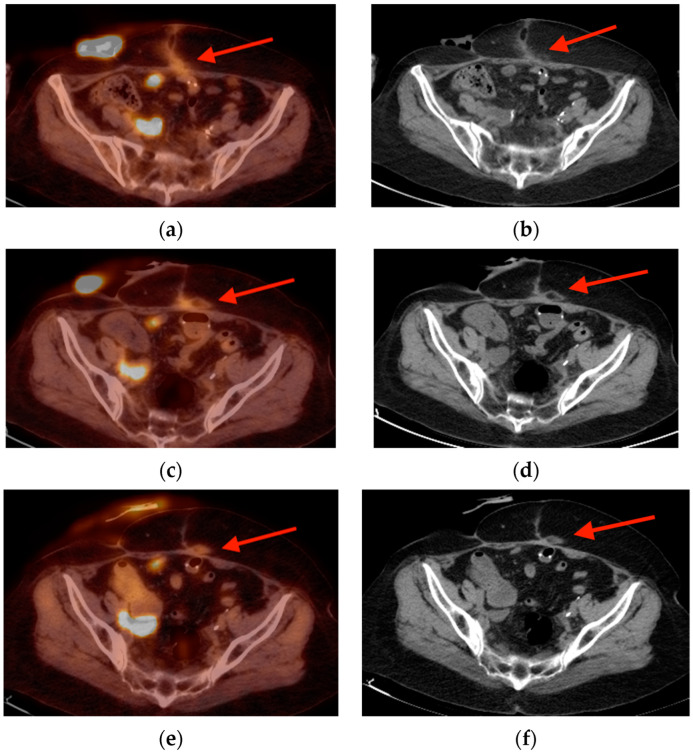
Multiple scans were obtained in a 72-year-old woman with bladder cancer after she had undergone surgical resection and reconstruction. Axial fused FDG PET/CT and non-fused CT images obtained at time 0 (a,b), 6 months later (c,d), and 12 months later (e,f) show evolution of a focal area of radiotracer uptake within the soft tissues of and anterior abdominal wall along the surgical scar, with macroscopic areas of fat (red arrows). CT = computed tomography. FDG PET/CT = fluorine-18 fluorodeoxyglucose positron emission tomography/computed tomography.
